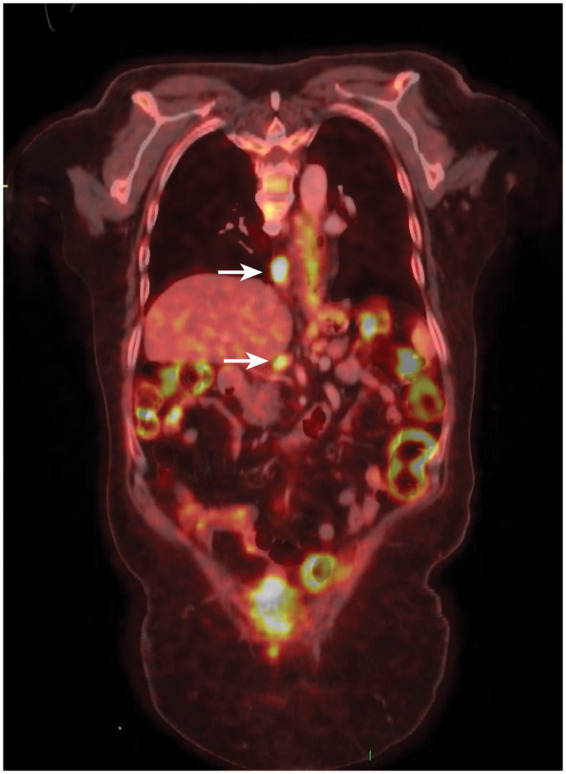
A restaging positron emission/computed tomography scan showing 2 enlarged paraoesophageal and coeliac lymph nodes (white arrows).
